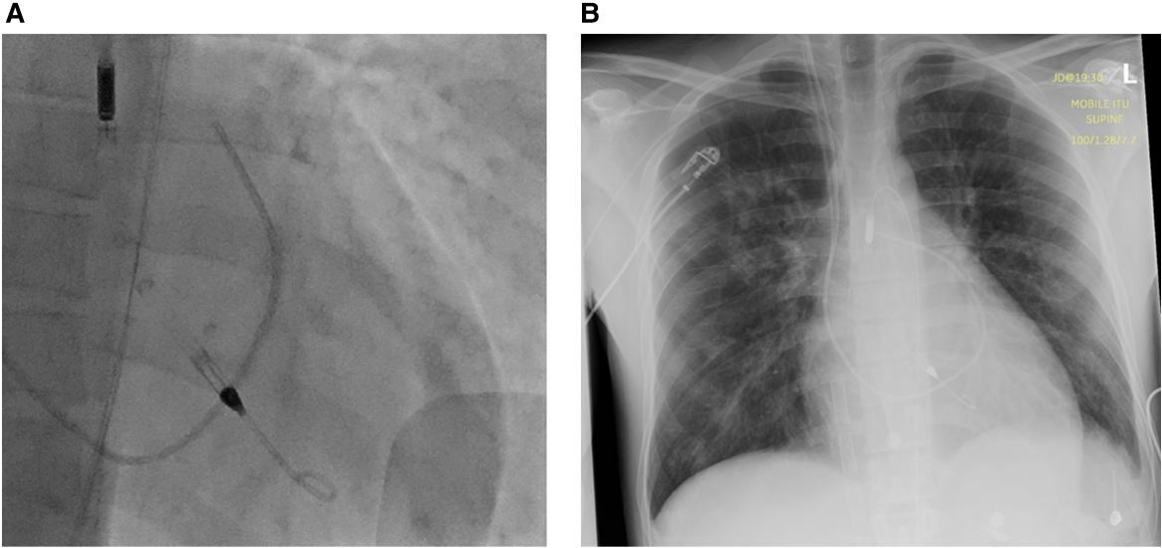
(*A*) Fluoroscopy antero-posterior view. Impella in correct position into the left ventricle (LV). (*B*) Postero-anterior chest X-ray. A 25 Fr multistage VA-ECMO venous cannula from the inferior vena cava with the tip at the level of the right atrium. Impella in correct position into the LV.
